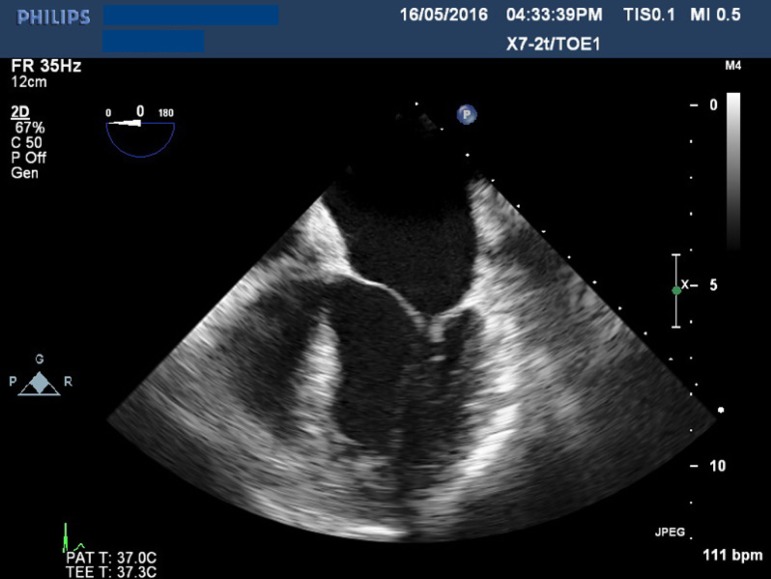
Transesophageal echocardiogram showing severely thickened mitral valve
